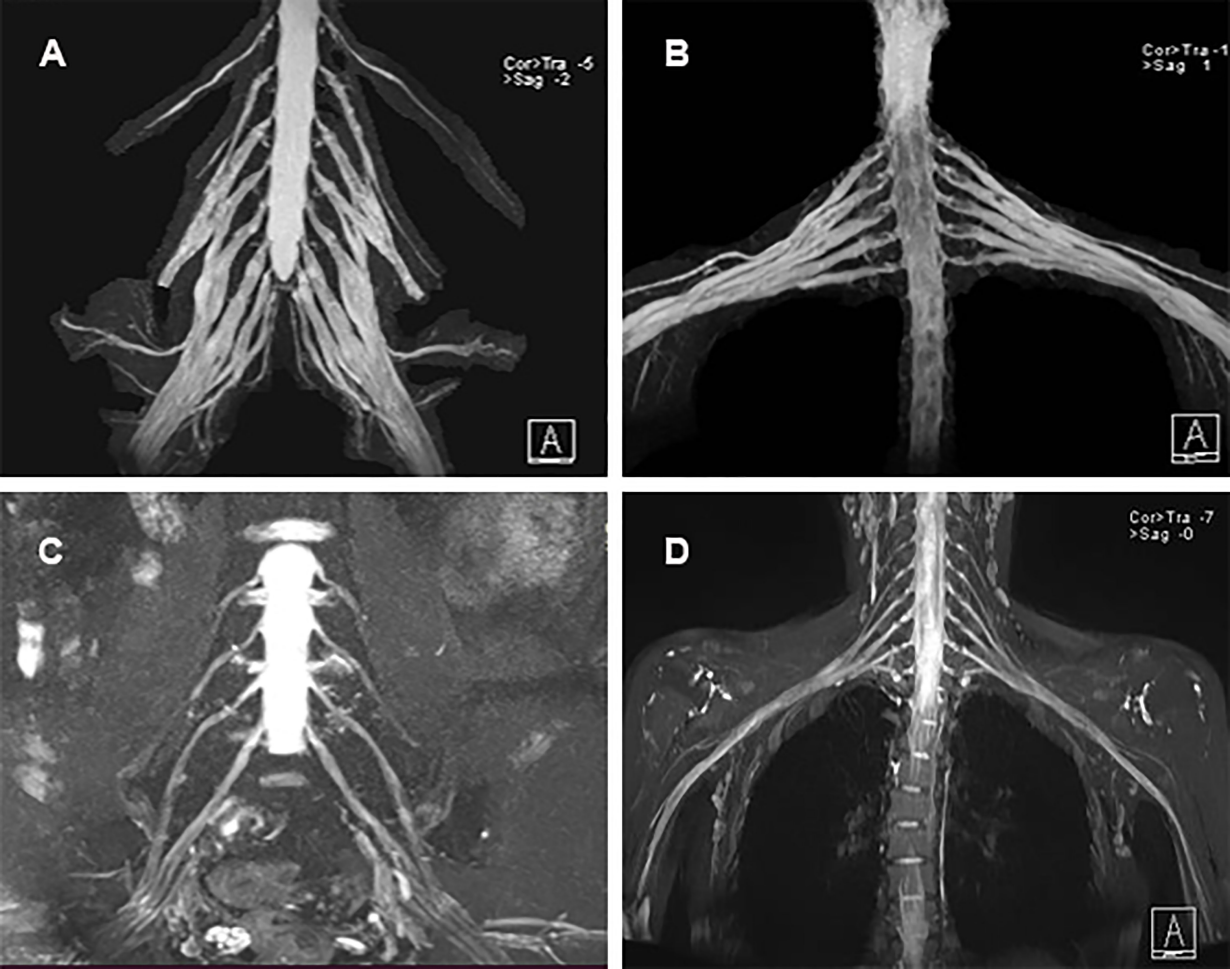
MRI neurography findings. (A) Diffuse thickening of the cervical roots; (B) Diffuse thickening of the lumbosacral nerve roots; (C, D) Normal cervical roots and lumbosacral nerve roots of healthy control.
